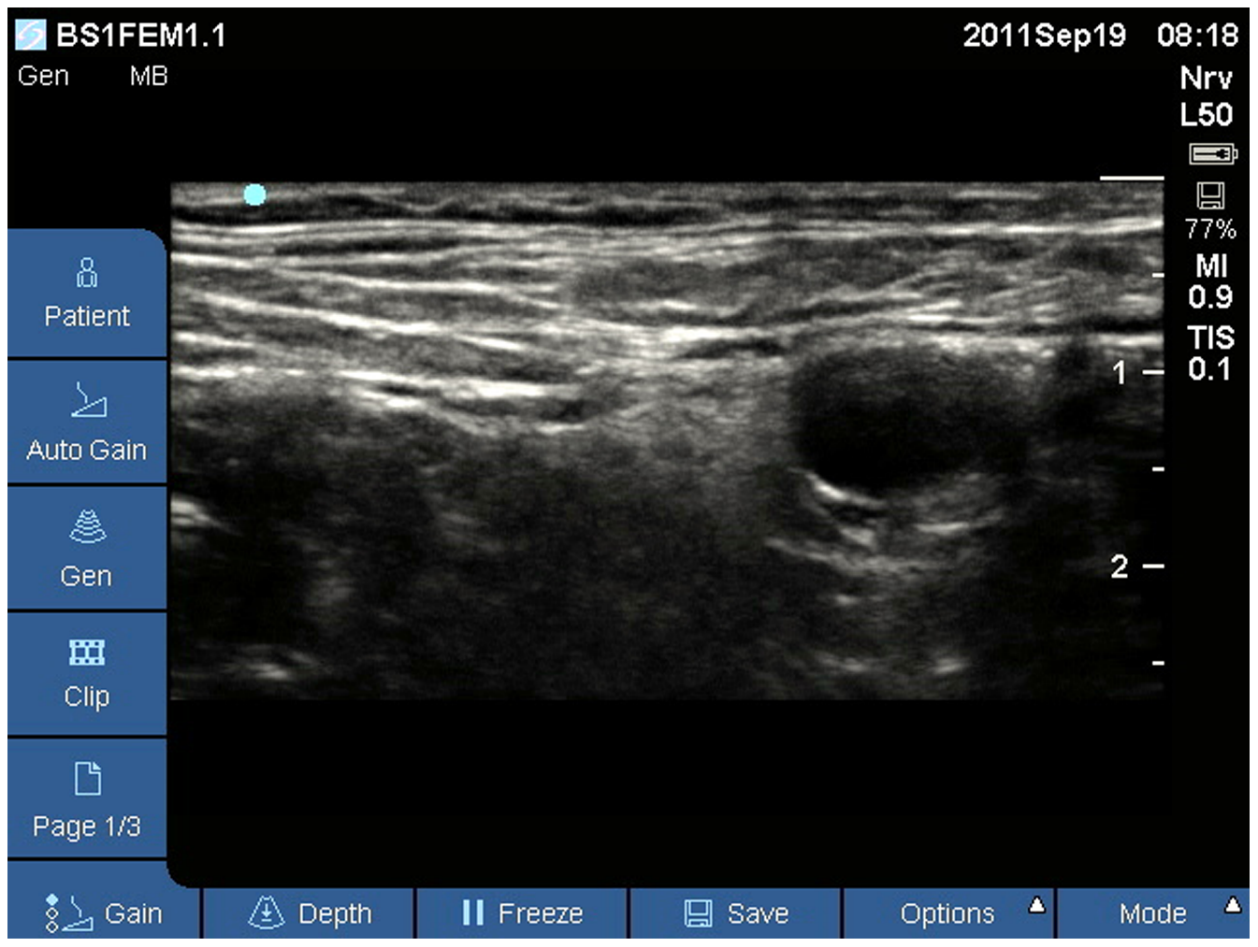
US picture showing a femoral nerve used in substudy #2. This ultrasound image is an example where gross identification of a large nerve was reduced by both expert and trainee observers.
